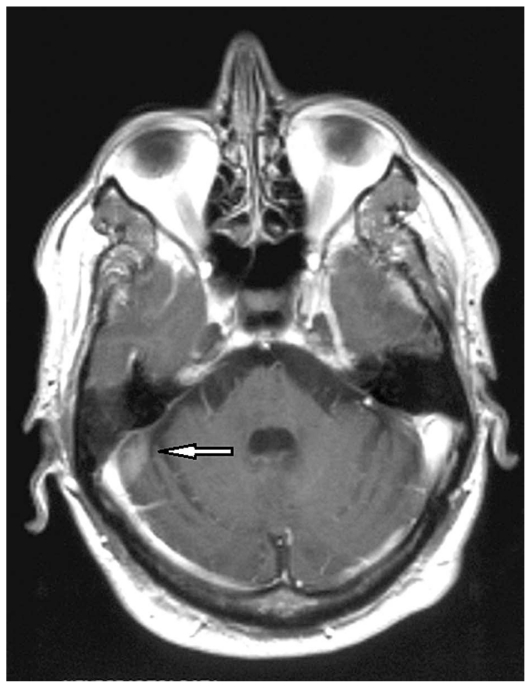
Neuro-sarcoidosis (T1 MRI sequence): homogeneous contrast enhancement of the leptomeninges and of a granuloma located in the posterior fossa.
